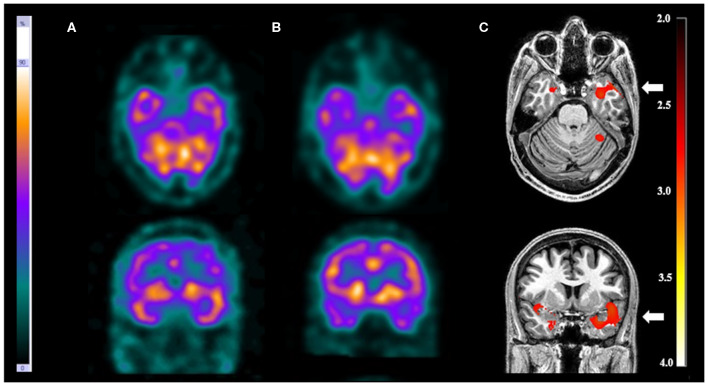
Patient #5 had pharmacoresistant epilepsy. Axial sections on first line and coronal sections on second line of (A) ictal SPECT and (B) interictal SPECT visual analysis showed a possible epileptogenic zone in the left temporal lobe. (C) SISCOM performed with SPM confirmed a focal area of hyperperfusion in the left temporal lobe (arrow). The reference standard suggested left temporal lobe epilepsy.
